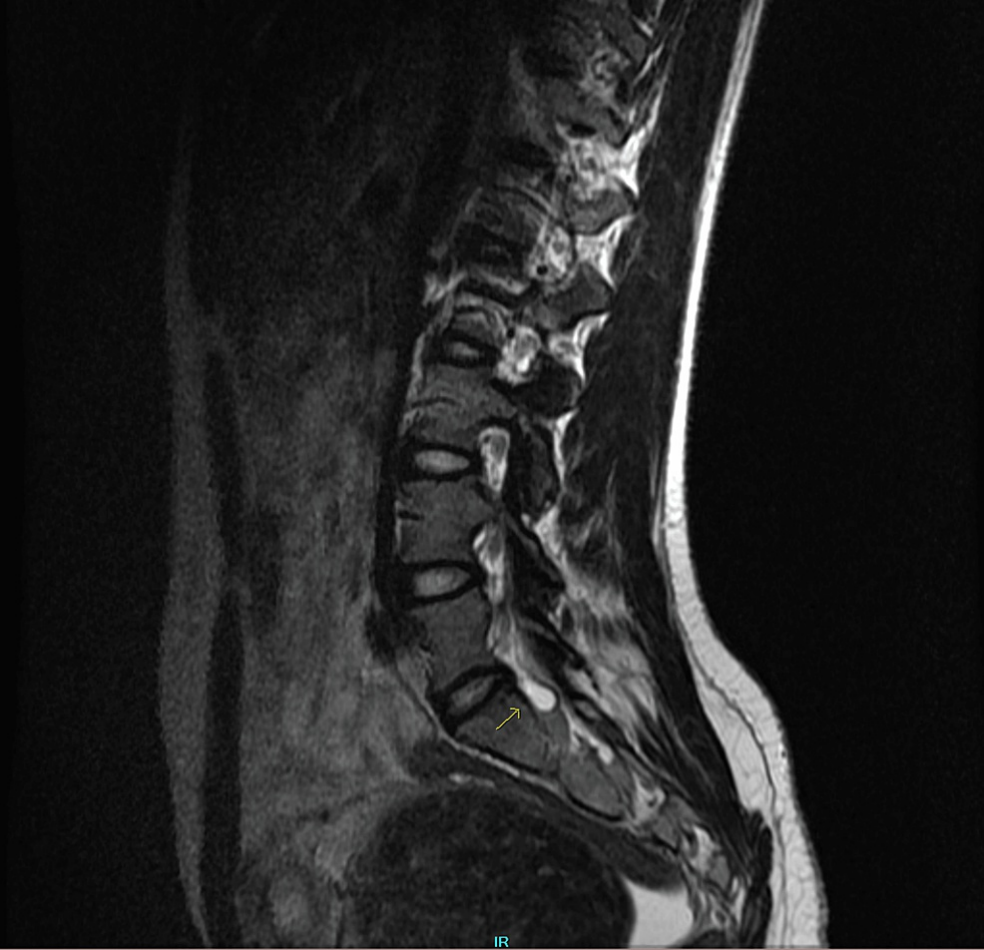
Sagittal lumbar MRI showing a tiny Tarlov cyst indicated by an arrow.
